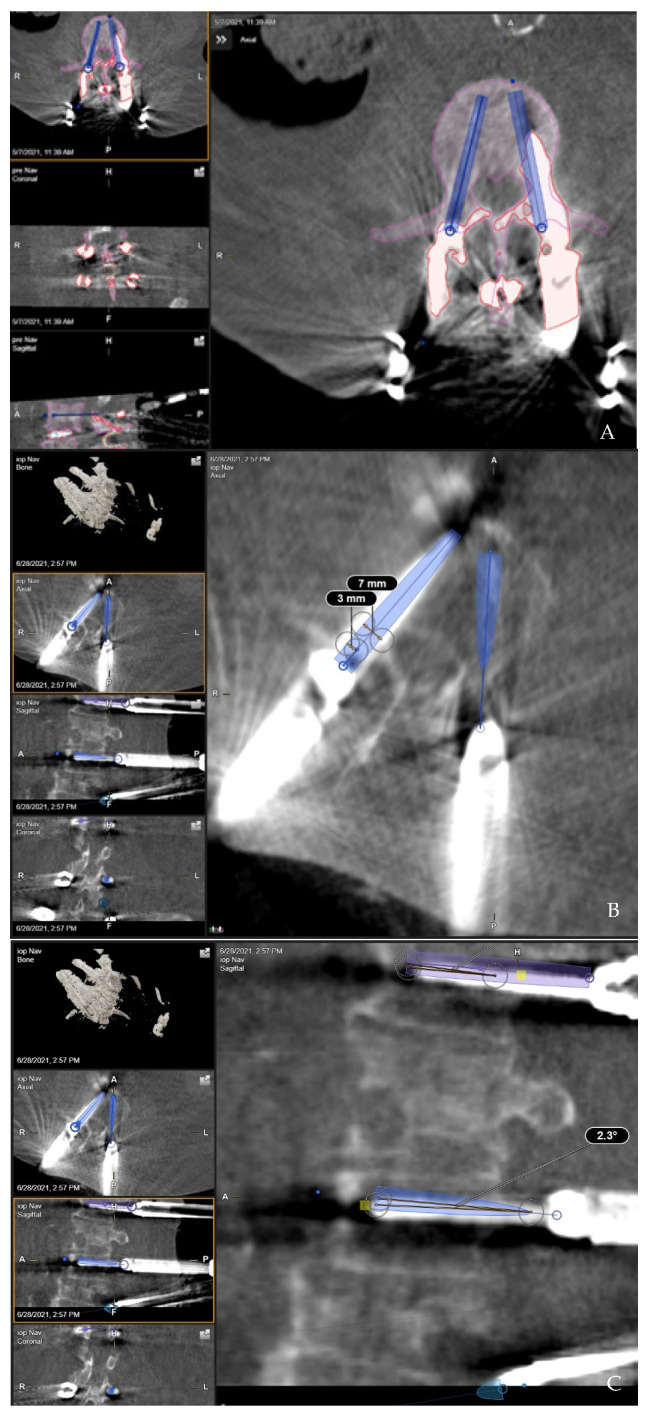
Accuracy of the implanted pedicle screws and deviation compared to the preoperatively planned screw trajectories were measured using the image overlay technique following rigid and elastic fusion of the preoperative CT scan, registration iCT scan and control iCT scan. (A). Implanted screws are segmented in red and the trajectory of the preplanned screws in blue. Entry point and tip point deviation were measured in the axial plane. (B). Overlay of a preplanned screw trajectory with the implanted pedicle screw. Entry point deviation 3 mm to medial and lateral from the entry point of the preplanned screw trajectory was considered acceptable for correct pedicle screw placement. (C). Angular deviation was measured in the lateral plane via the angular measurement tool in the BrainLab software using the measurement of the angle between the axis of the implanted screw and the preplanned screw trajectory.
